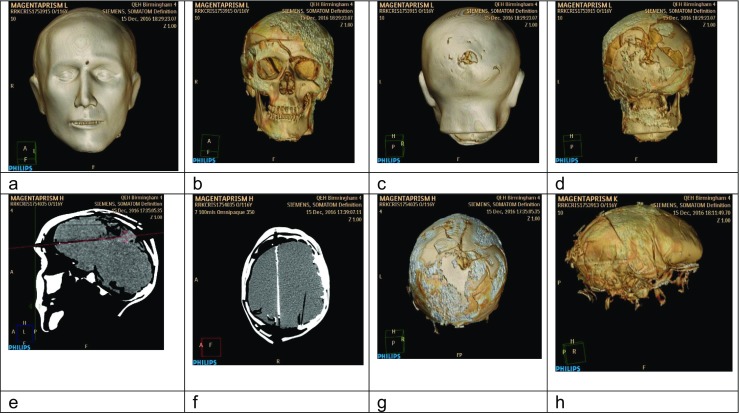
CT reconstruction images from Phillips Brilliance Extended Work Station; a–d show Face 6/Lima. a Entry wound. b Fracture patterns underlying entry site. c Exit wound. d Fracture patterns under exit site. e–g Face 2 /Hotel. e Sagittal view of bullet trajectory with fractures and exit wound. f Cross-sectional view of same features; OmnipaqueTM300 contrast present in the bullet tract. g 3D reconstruction of fractures. h Face 5/Kilo; 3D reconstruction of gelatine brain with posterior damage post shot.
